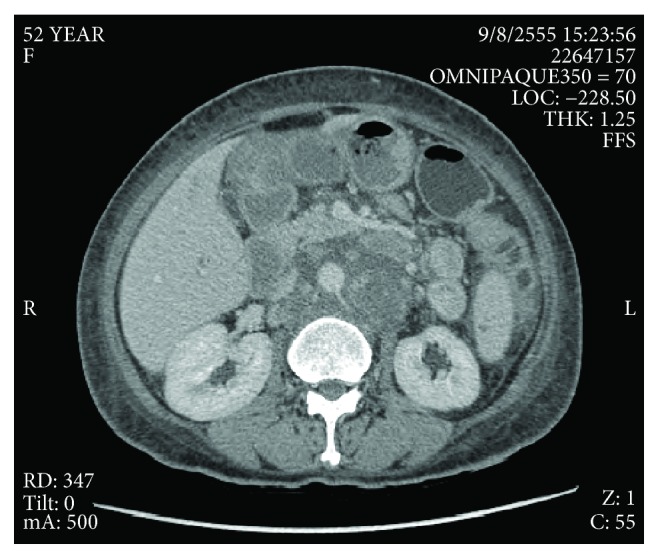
Abdominal CT scan at the time of diagnosis demonstrated matted paraaortic nodes.
